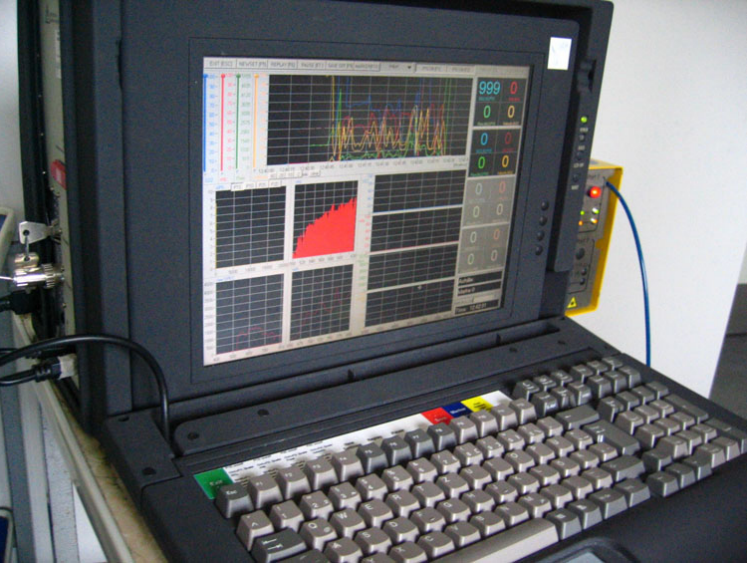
Oxygen-to-see system combining Laser Doppler flowmetry and spectrophotometry non-invasively to determine tendon capillary blood flow, tendon oxygen saturation, and tendon postcapillary venous filling pressures.
